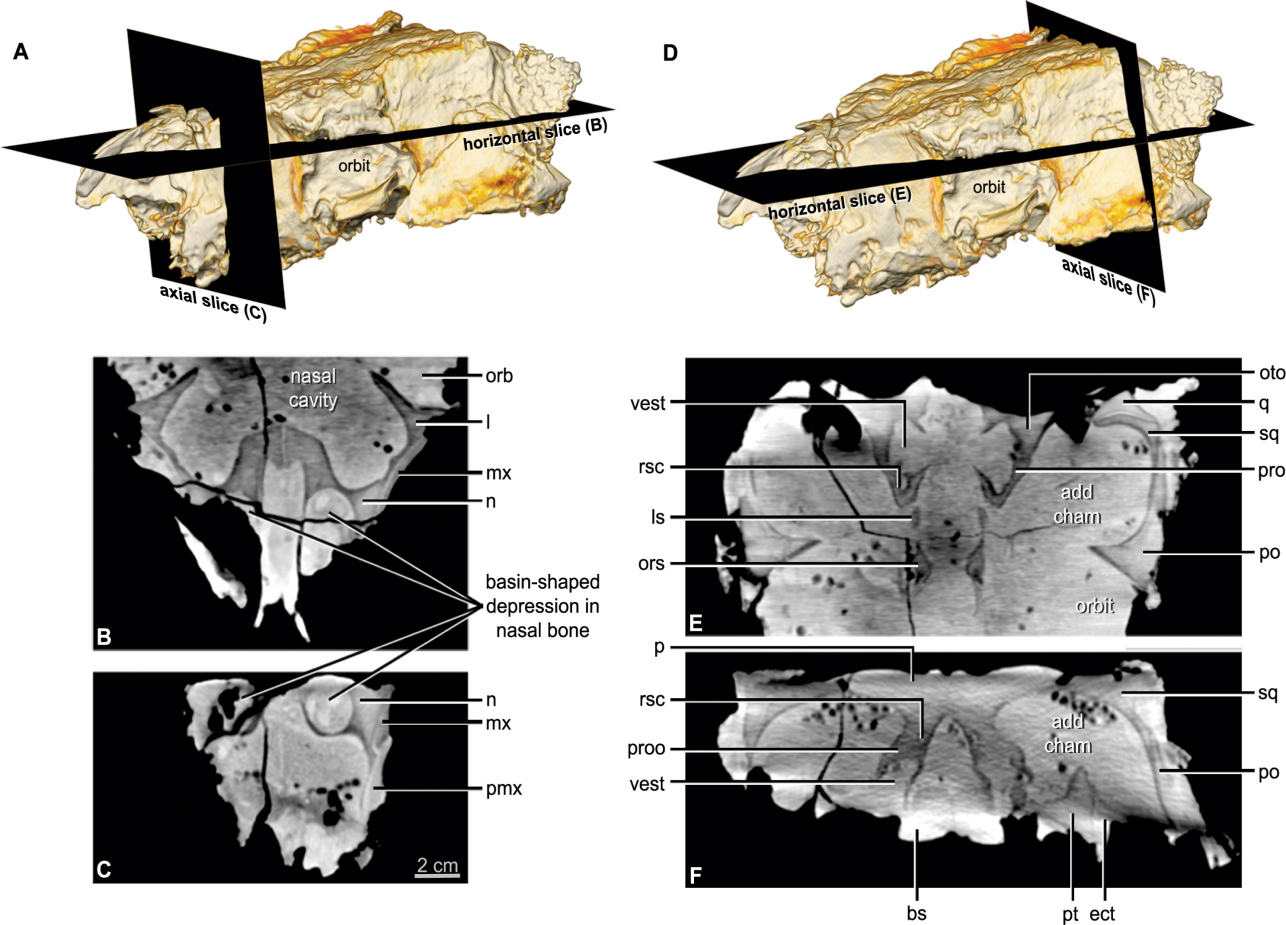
CT scan slices through the nasal cavity A, B, C, and braincase D, E, F regions of *Kunbarrasaurus ieversi* gen. et sp. nov. (formerly *Minmi* sp.) (QM F18101). (A, D) Volume rendering of the entire skull showing the positions of the horizontal slices in (B, E) and the axial (vertical) slice in (C, F). Abbreviations: ls, laterosphenoid; ors, orbitospenoid; oto, otoccipital; proo, prootic; rsc, rostral semicircular canal; vest, vestibule. For other abbreviations see Fig. 1. Scale bar equals 2 cm.
